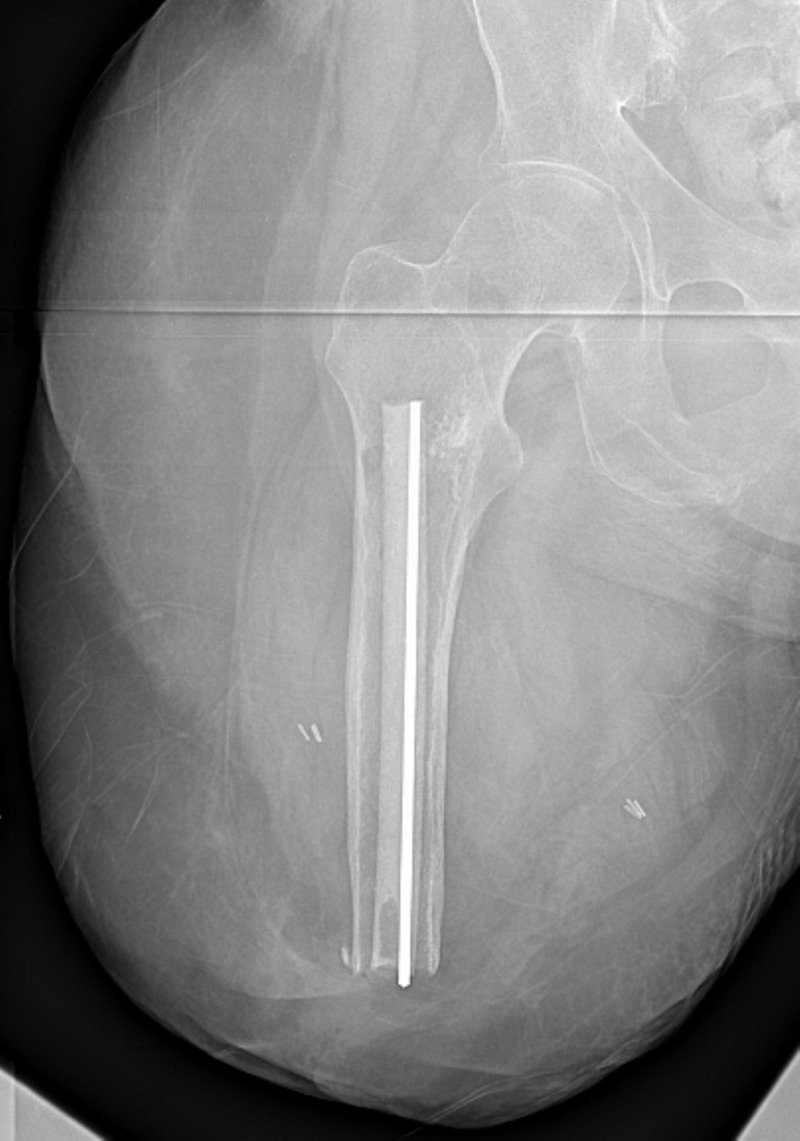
Temporary antibiotic spacer. Right femur anterior-posterior radiograph with temporary spacer containing 2 g vancomycin and 3.4 g tobramycin mixed with 40 g cement. The central metal pin aids in the preparation of the spacer and also with eventual removal.
